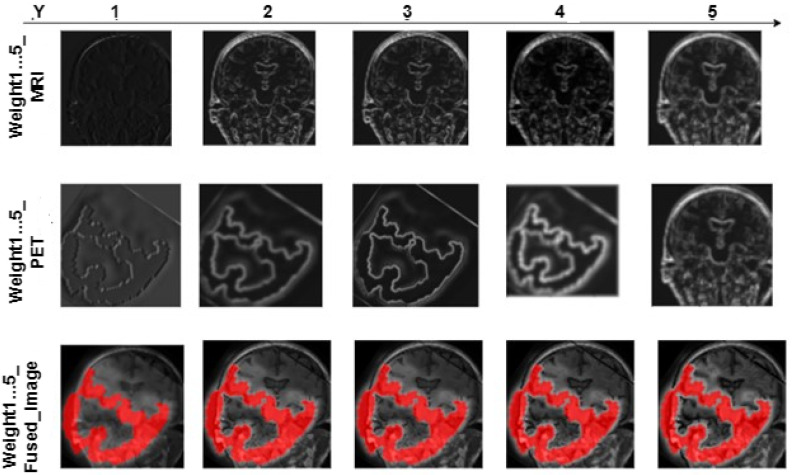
MRI-PET Fusion weights and results compared to feature depth using VGG19 without Transposed Convolution.
